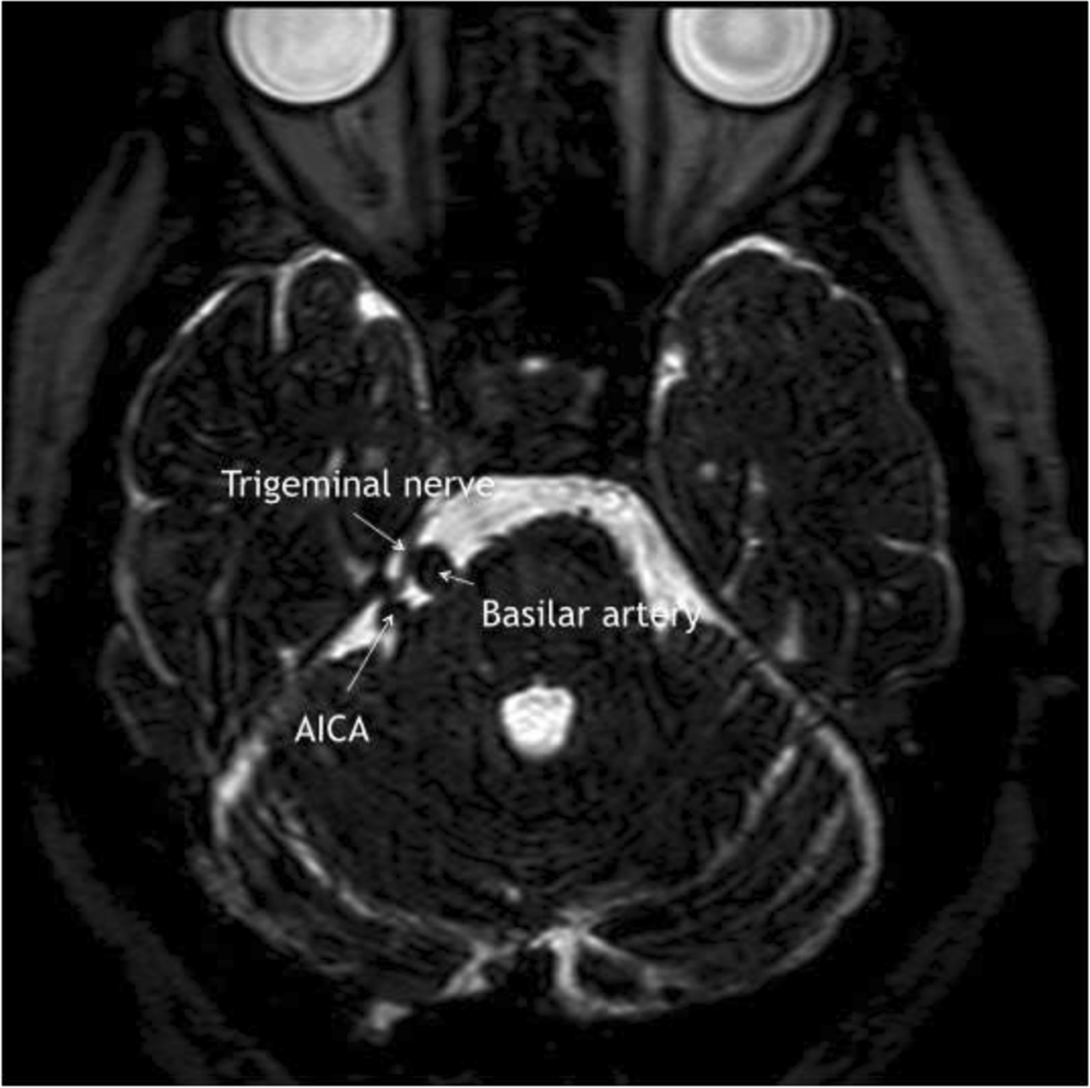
MRI showing right-sided neurovascular contact between the basilar artery and the trigeminal nerve. While it may look like the anterior inferior cerebellar artery (AICA) is in fact also compressing the trigeminal nerve, the treating neurosurgeon (PR) did not find this to be the case during surgery.
